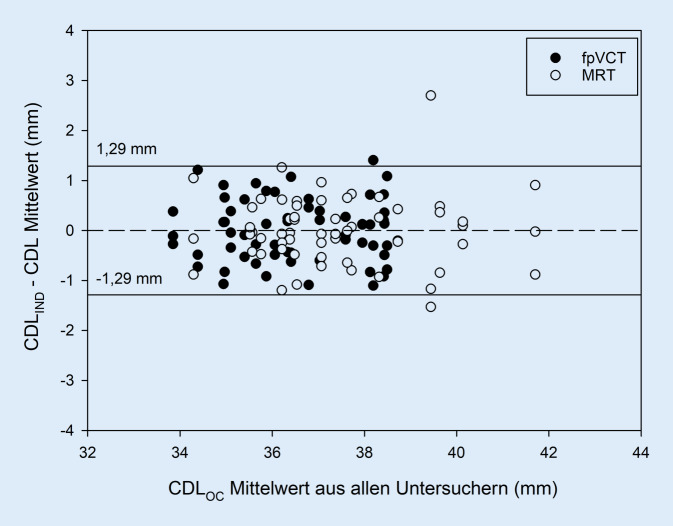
Die einfaktorielle Varianzanalyse mittels ANOVA in Bezug auf die Bildgebungsmodalität zeigte einen signifikanten Haupteffekt: F (1, 105) = 20,70; *p* = 0,000015. Dabei waren die vermessenen Werte der CDL in der MRT größer als in der CT (*M* Differenz = 0,89 mm; 95%-Konfidenzintervall 0,50–1,28). Unsere Analyse der Untersucher zeigte keinen signifikanten Effekt: F (2, 105) = 0,84; *p* = 0,437. Auch die Untersucher × Bildgebung-Interaktion zeigte keine Signifikanz: F (2, 105) = 0,342; *p* = 0,711. Die Unterschiede zwischen den Messungen der verschiedenen Untersucher waren für beide Bildgebungsmodalitäten klein und weder statistisch noch klinisch signifikant (*M* Differenz < 0,5 mm).
